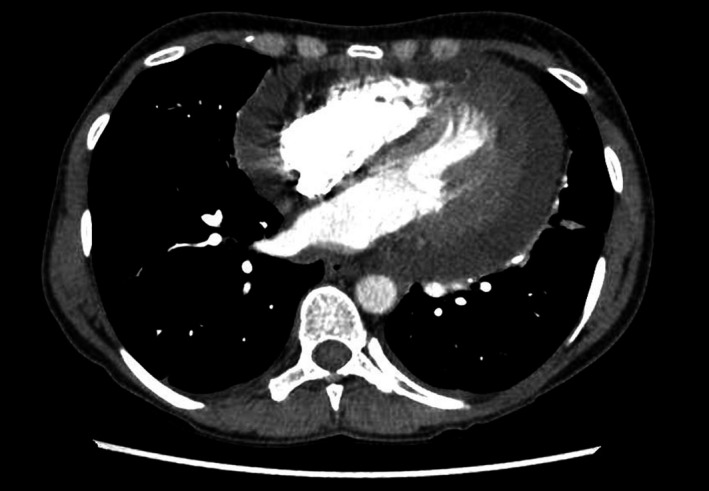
CT chest revealed a large pericardial effusion
